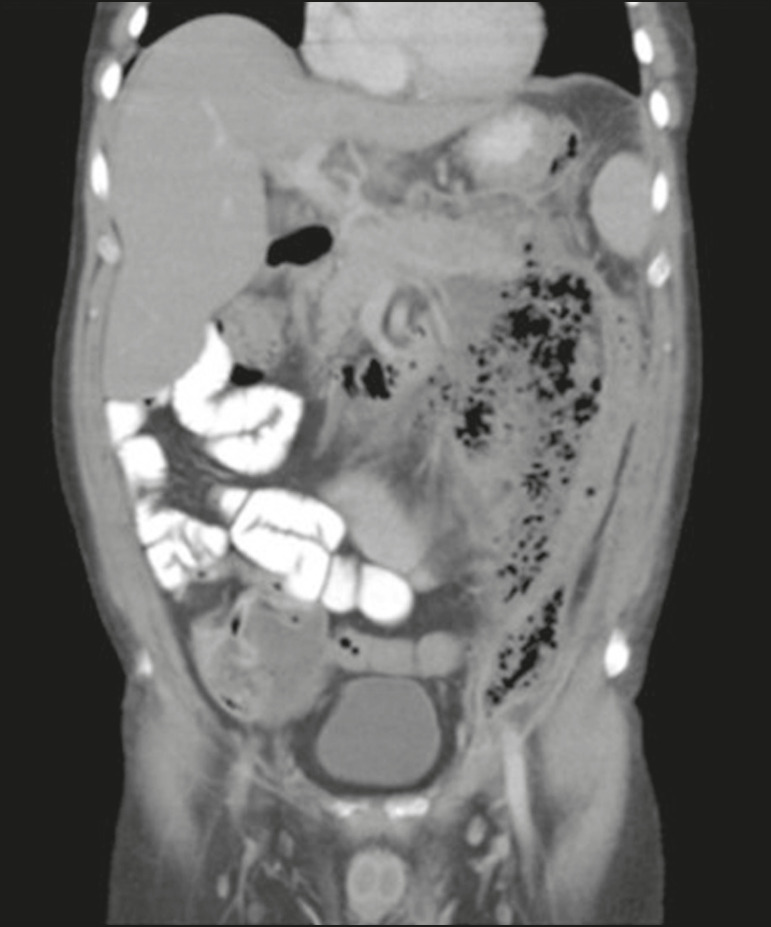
A 45 year-old male with hypertriglyceridemia and alcohol use disorder. Oral contrast-enhanced coronal CT, in the portal phase, showing acute pancreatitis complicated by an abscess invading the left inguinal canal.
